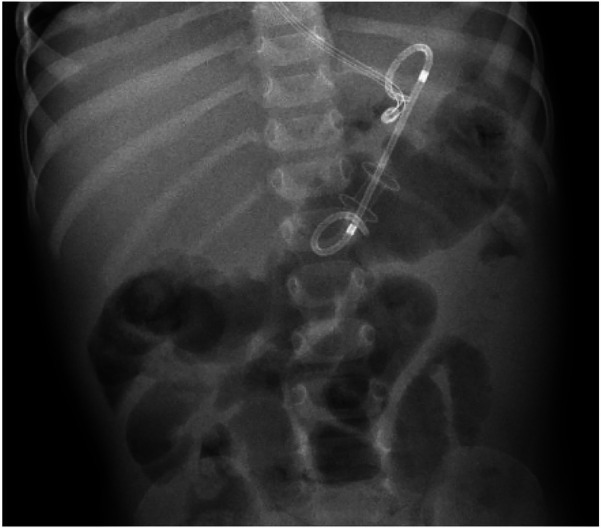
X-ray showing stent and pigtail.
